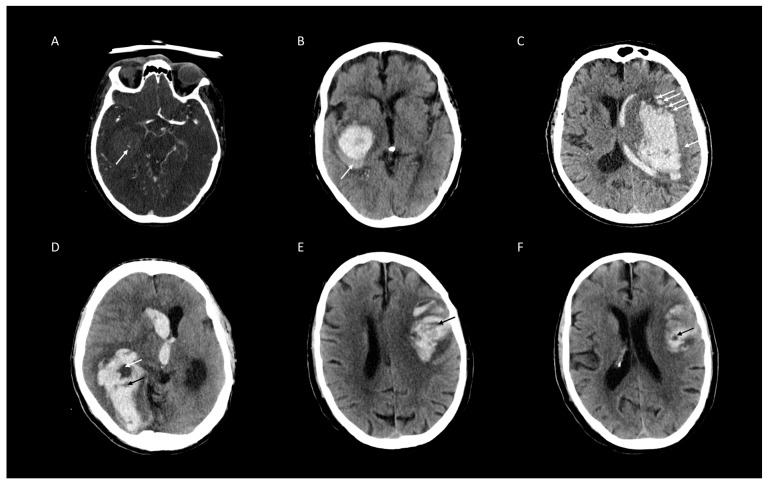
Representative examples of agreed ratings of four non-contrast computed tomographic (NCCT) markers and Spot Sign (SS) on CT-angiography (CTA) for intracerebral hemorrhage expansion. (A) SS on CTA (white arrow). (B) Blend Sign (white arrow). (C) Island sign (all white arrows). (D) Black Hole Sign (white arrow), Hypodensities (black arrow). (E) Hypodensities (black arrow). (F) Black Hole Sign (white arrow).
